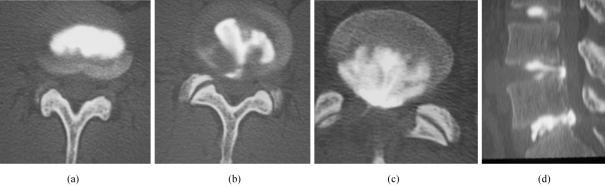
CT discographic patterns in a 36-year-old man who had low back pain with right buttock pain and right leg radiation. (a) Axial CT image shows a normal L3/4 disc (DDD grade 0). (b) Axial CT image shows a small L4/5 posterior annular tear (DDD grade 3). (c) Axial CT image shows extensive L5/S1 posterior annular disruption (DDD grade 4). (d) Sagittal recontructed CT image provides a good overview of a normal L3/4 disc, and posterior tears of protruding L4/5 and L5/S1 discs. Both lower discs were symptomatic.
